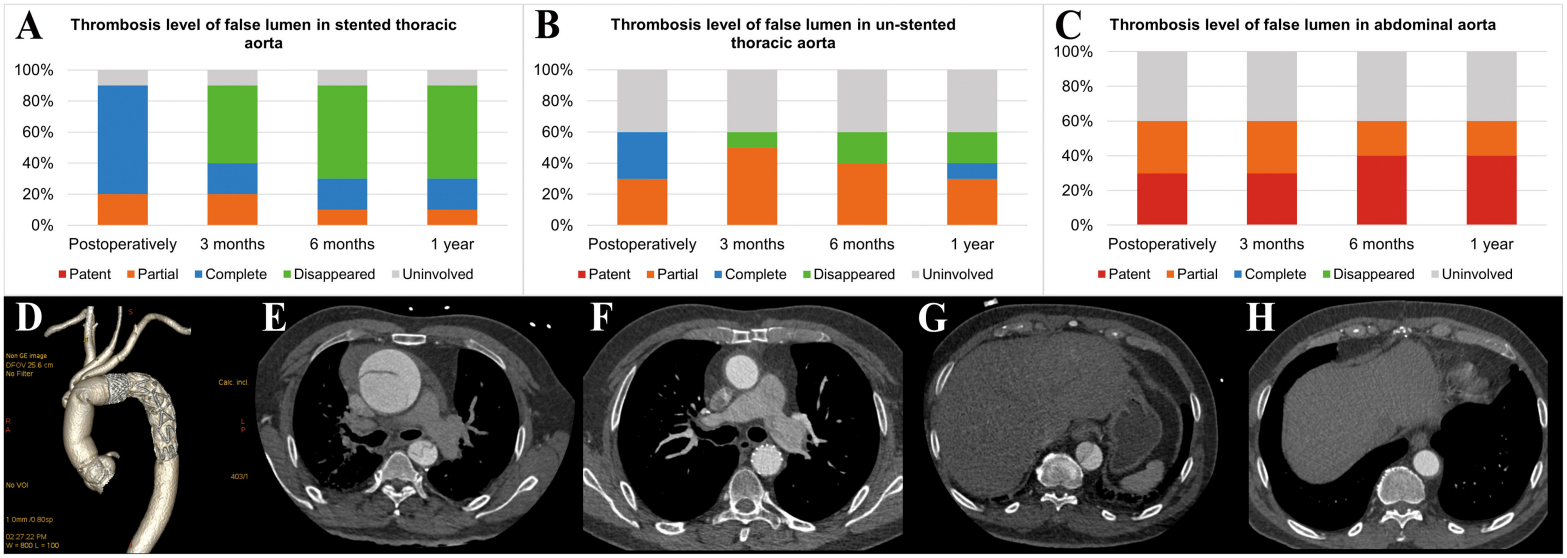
Aortic remodeling after surgery. (A) Proportions of different FL thrombosis levels in the stented thoracic aorta at different time points after surgery. (B) Proportions of different FL thrombosis levels in the unstented thoracic aorta at different time points after surgery. (C) Proportions of different FL thrombosis levels in the abdominal aorta at different time points after surgery. (D) The 3D reconstruction of aortic CTA shows the appearance of the SIS graft prosthesis after AAR+TAR+FET implantation surgery. (E–H) The axial view of aortic CTA presents comparisons of the aorta preoperatively and 3 months postoperatively in the same patient who underwent Bentall+AAR+TAR+FET implantation. (E) At the pulmonary artery bifurcation level, the intimal flap, TL and FL could be seen clearly in the ascending aorta and descending aorta preoperatively. (F) The ascending aorta was replaced with a terylene vascular graft, and the FL completely disappeared after FET implantation. (G) At the T10 level, the intimal flap, TL and FL could be seen clearly in the descending aorta preoperatively. (H) The FL disappeared in the unstented region by 3 months postoperatively. FL, false lumen; 3D, three-dimensional; CTA, computed tomography angiography; AAR, ascending aorta replacement; TAR, total arch replacement; FET, frozen elephant trunk; TL, true lumen.
